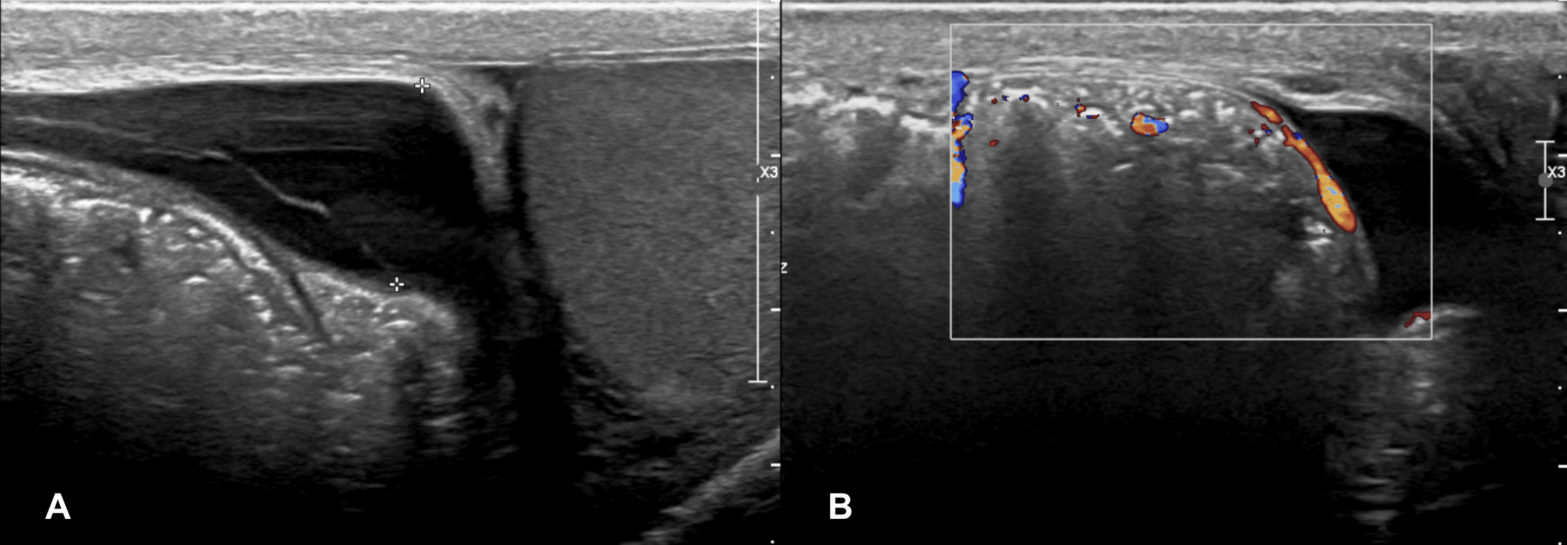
A) Ultrasound showing the right inguinoscortal hernia containing bowel loops, B) Color Doppler showing the viability of the bowel
